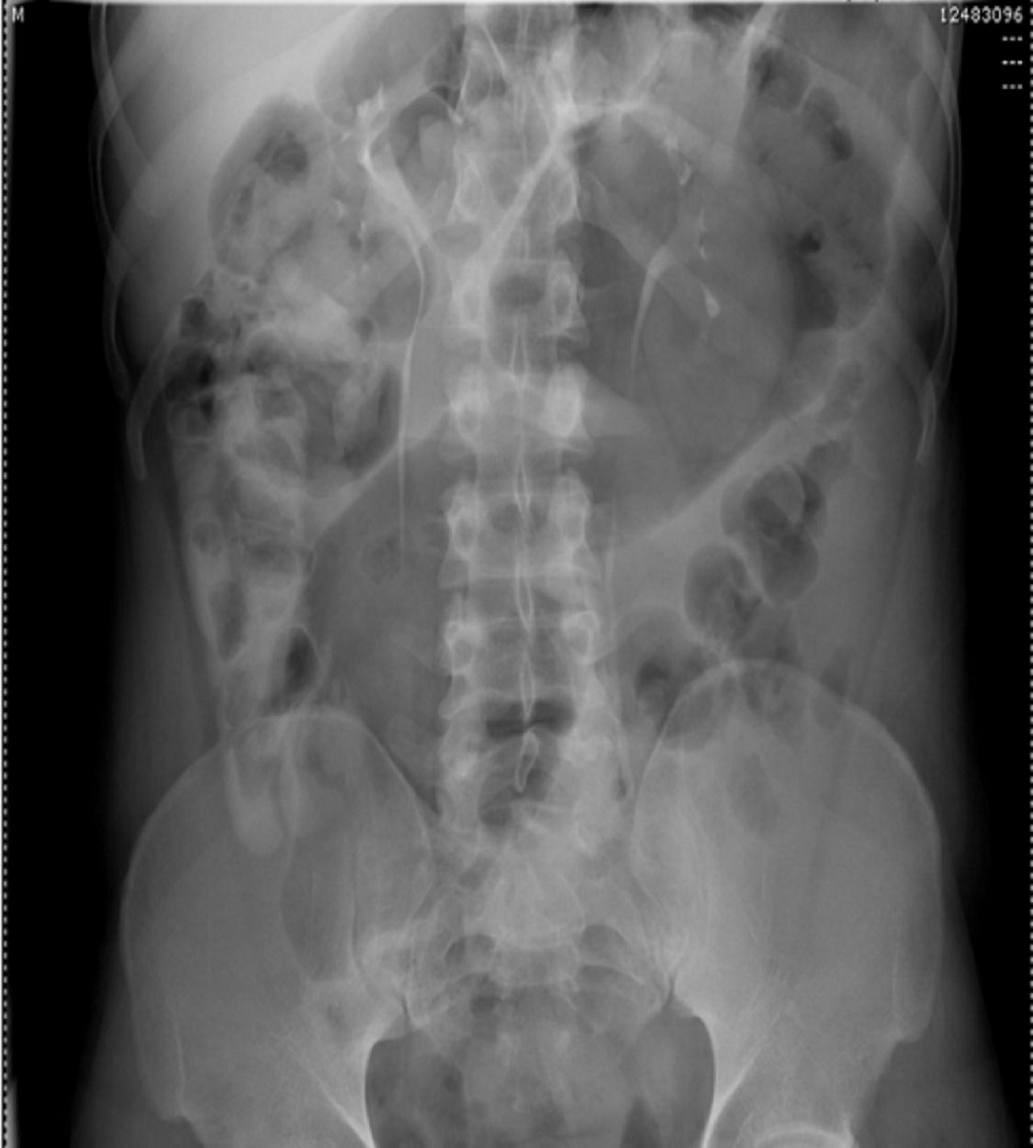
Plain Film Radiograph Anteroposterior view abdominal X-ray demonstrating marked dilatation of the sigmoid colon.
